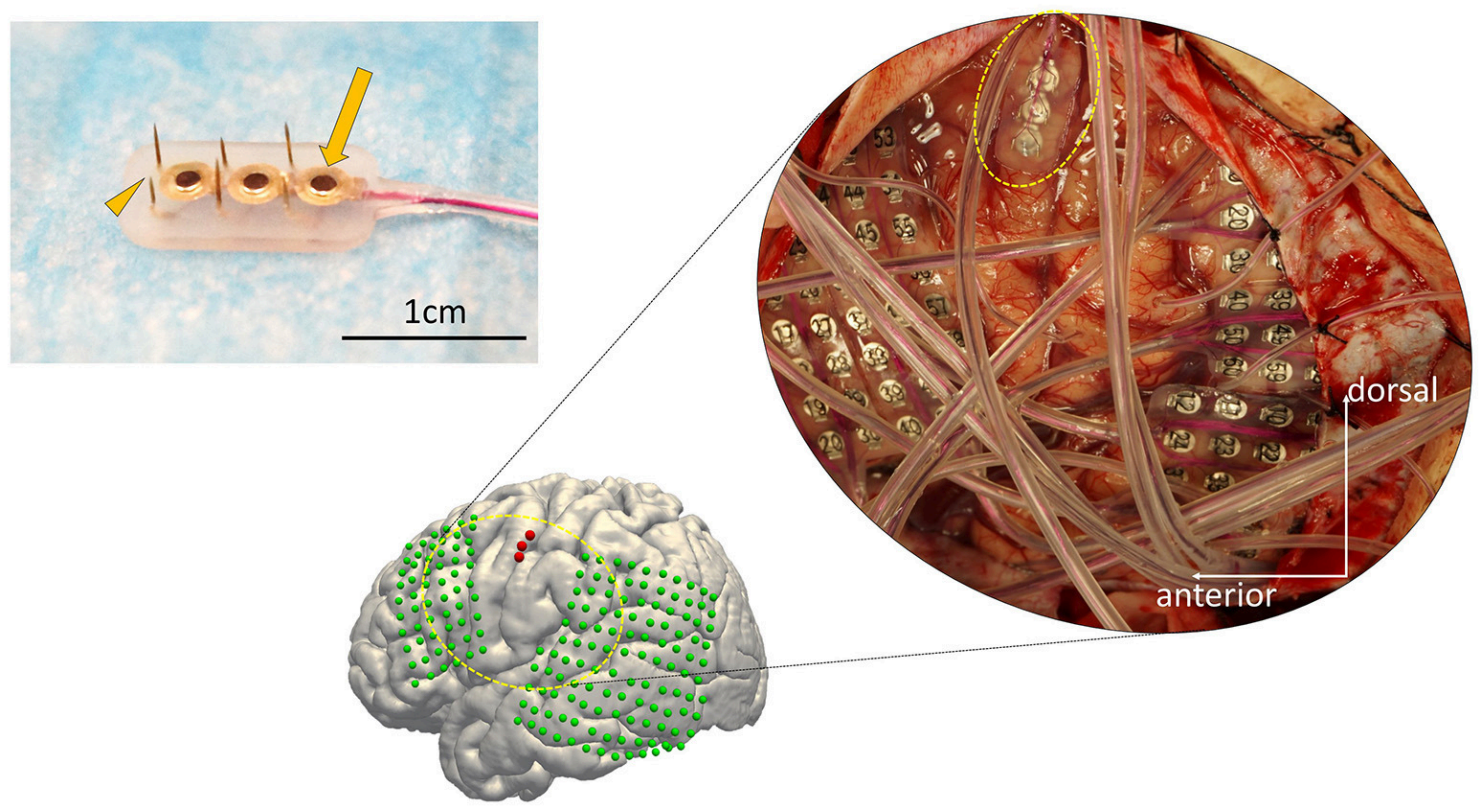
The configuration of the hybrid electrode and an example of implantation. (Left) The electrode fabricated for this study (7 × 13 mm) consists of three grid electrodes (macroelectrodes, arrow) and six needle electrodes (microelectrodes, arrowhead) on the side (Unique Medical Co., Tokyo, Japan). The surface diameter of each macroelectrode was 1.5 mm, whereas that of the microelectrodes was 200 μm. The length of the needles alternated between 1.5 and 2.5 mm to reduce the physical force needed for implantation. (Right) Implanted electrodes overlaid on a 3D reconstructed pre-operative MRI, displayed together with the intraoperative photograph. The hybrid electrode is encircled by the dotted yellow line in the intraoperative photograph. The subdural grid electrodes are shown in green. The hybrid electrode is shown in red.
